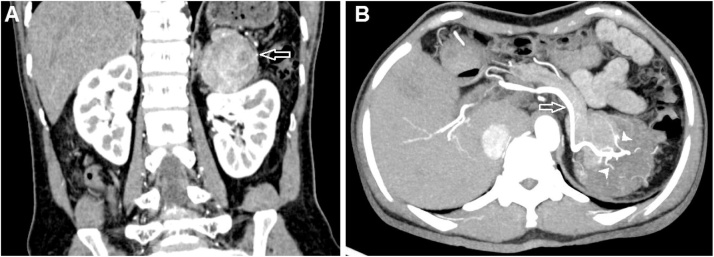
Coronal reconstruction of CT scan in the arterial phase (A) and axial maximum intensity projection in the arterial phase (B). The mass had well-defined borders and compressed the left kidney (A, arrow). The arteries feeding this mass (B, arrowhead) was a branch of the splenic artery (B, arrow).
